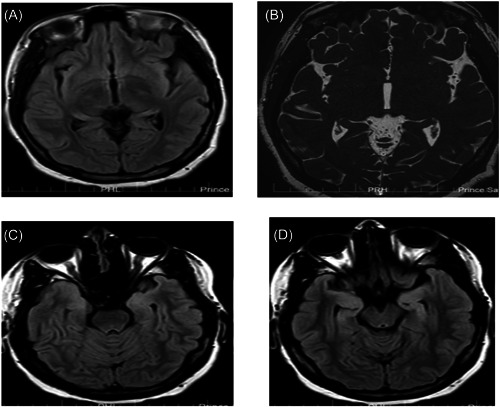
(A–D) MRI brain sections exhibiting prominent sulci, mainly of the Sylvian fissure, corpus callosum atrophy, and bilaterally prominent temporal horns with relatively symmetrical increased FLAIR signal intensities of both hippocampal formations. FLAIR, fluid‐attenuated inversion recovery; MRI, magnetic resonance imaging.
